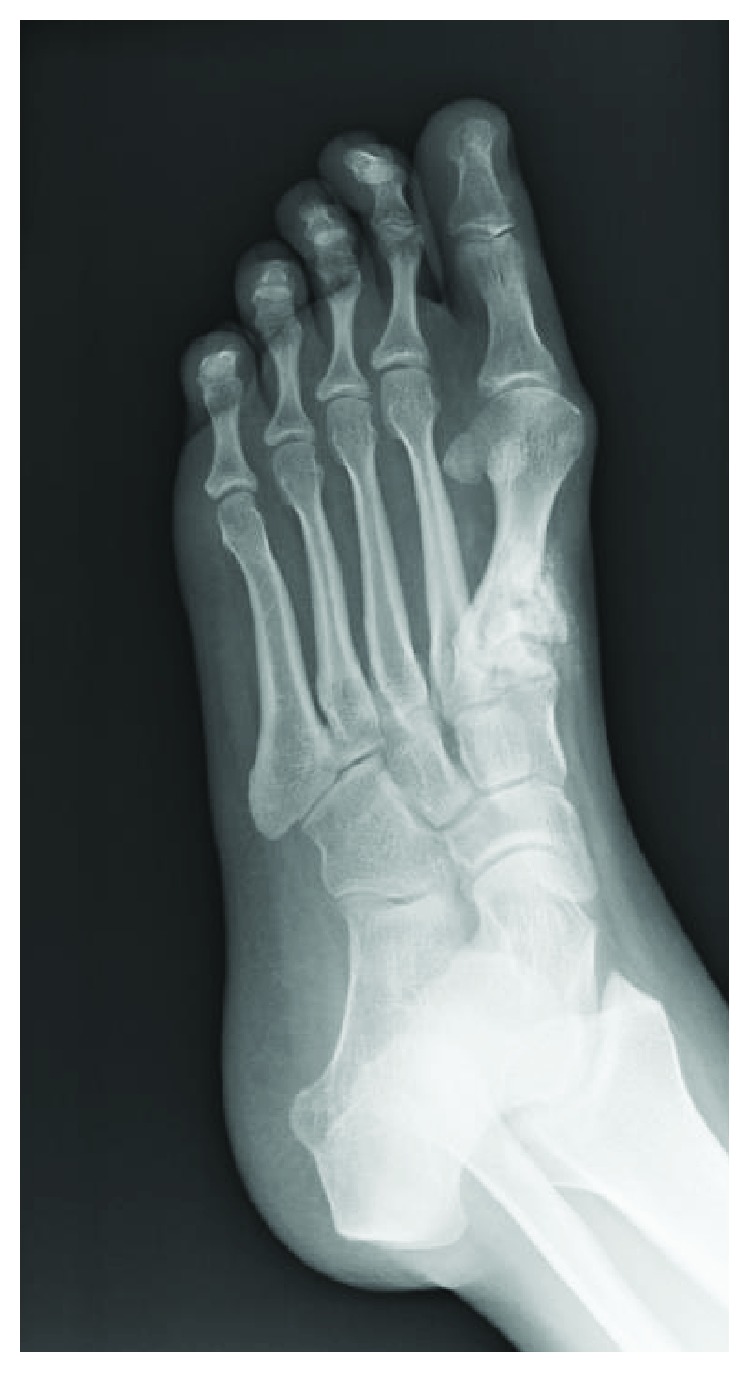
Plain radiographs of the left foot showing an expansile, radiolucent lesion of the first metatarsal base surrounding the known cement implantation of the first metatarsal.
